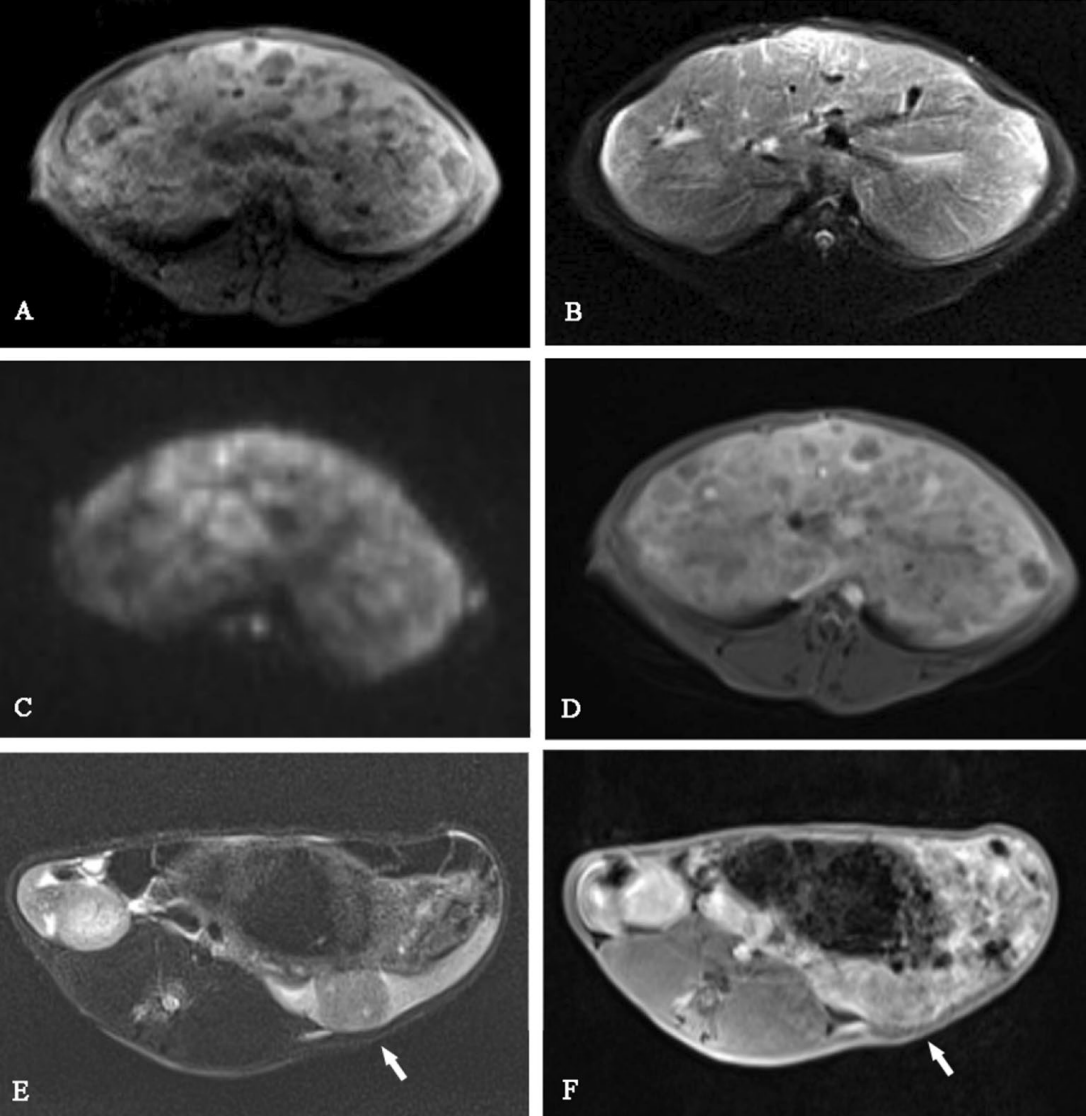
MRI manifestations of liver metastases were multiple nodular and punctate abnormal signal shadows in the liver, low signal on T1WI (A), slightly high or equal signal on T2WI (B), high signal on DWI (C), and circular enhancement on enhanced scanning (D); E, F: T2WI and T1WI sequences showed that the liver was enlarged and with a nodular shadow (white arrow).
